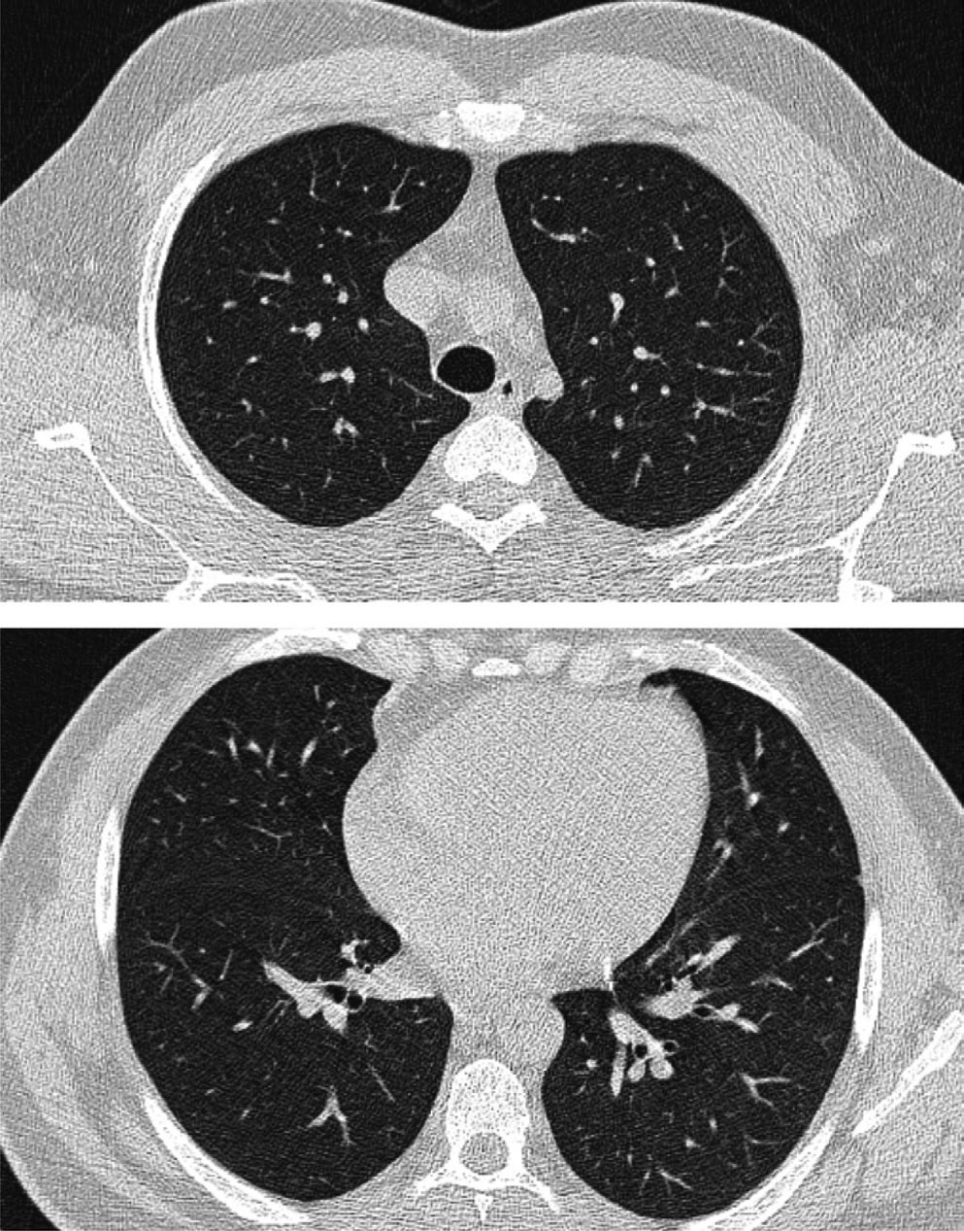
Two axial cuts of lung HRCT of the patient on day first of admission
